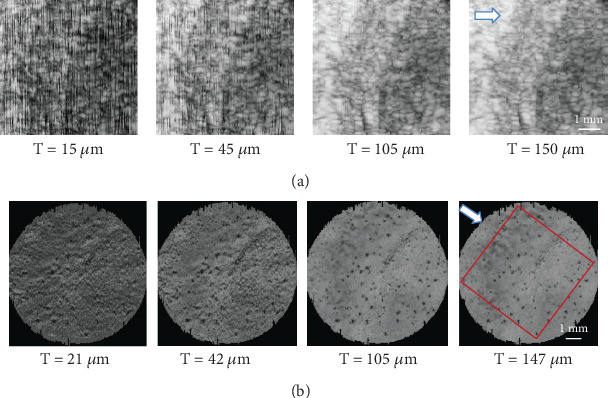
Typical en face images for the ultrasound and micro-CT imaging. (a) MIP images of the ultrasound imaging with different VOI thickness: 15, 45, 105, and 150 *μ*m, respectively. (b) MIP images of the micro-CT imaging with different VOI thickness: 21, 42, 105, and 147 *μ*m, respectively. The ultrasound scanning direction (arrow) is indicated in the last images of (a) and (b) for the reference of comparison. The rectangular box in (b) indicates the region of ultrasound imaging. The scale bar is 1 mm in length.
